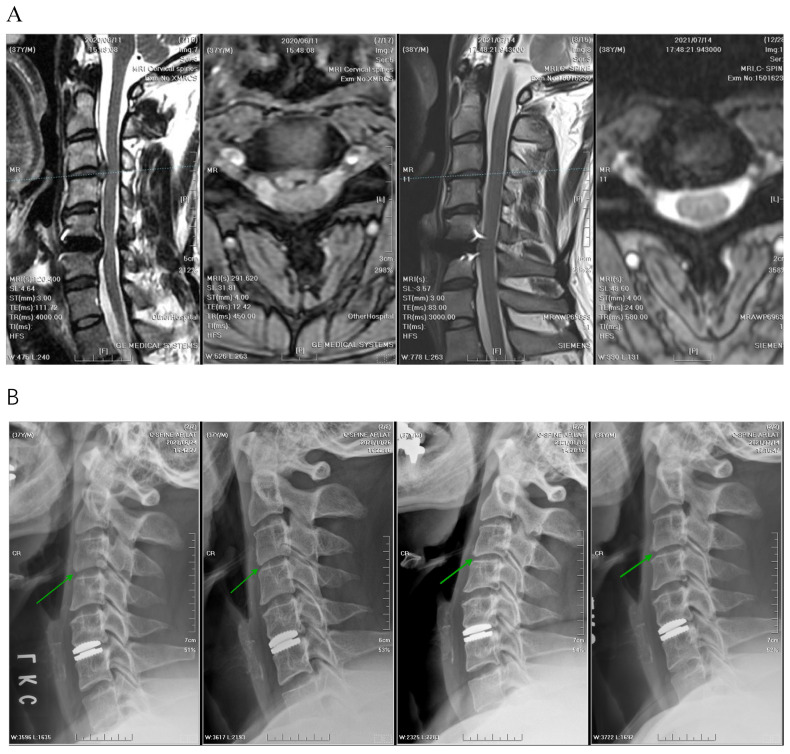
(A) The intervertebral disc height of C3–C4 decreased significantly from 5.0 mm to 3.2 mm before and after APECD (MRI before APECD on the left and one year after the surgery on the right). (B) The images from left to right are radiographs before APECD and 3 months, 6 months, and 1 year after the surgery. Green arrows point to the C3–C4 disc space. The reduction of disc height of C3–C4 was sufficient to be observed by the naked eye.
